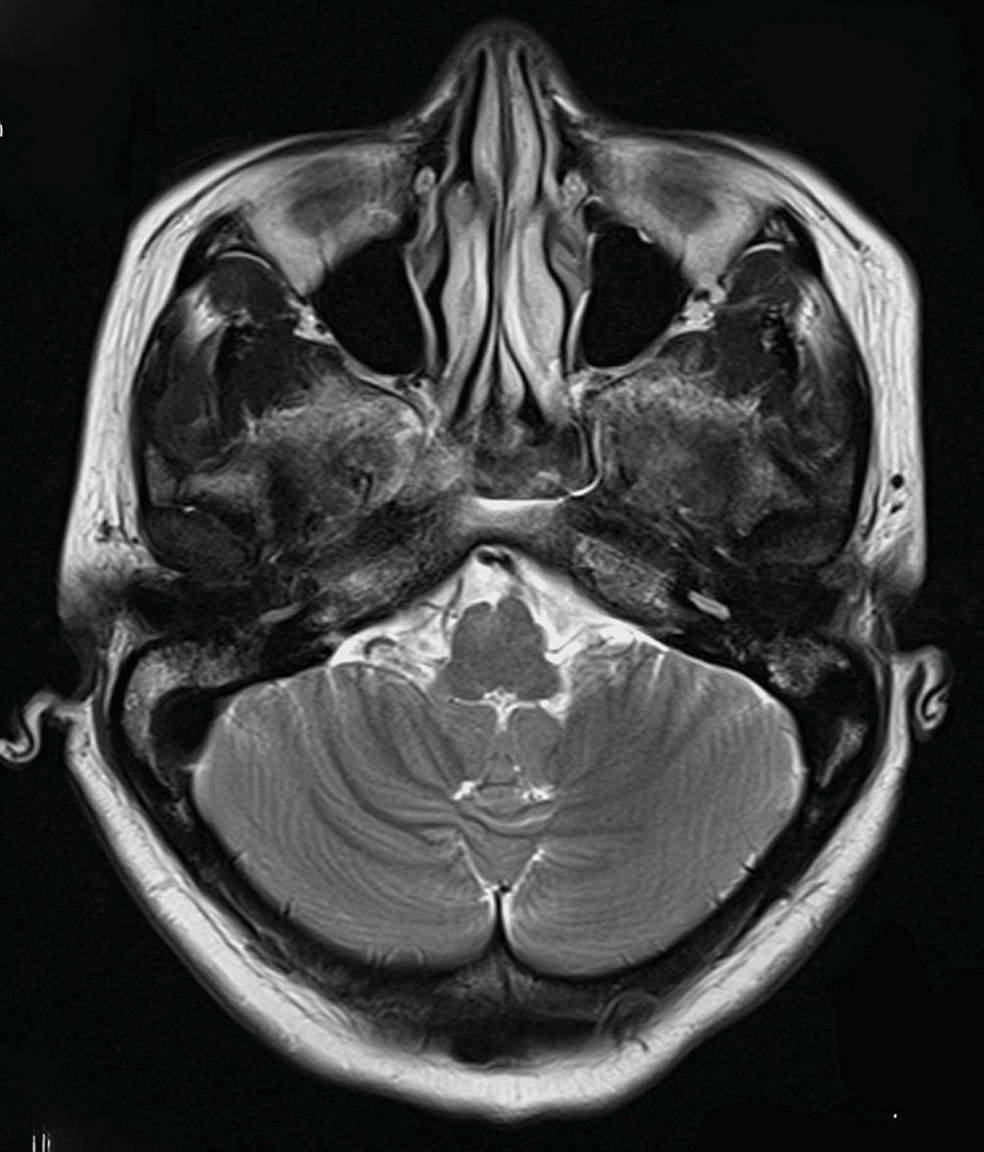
Follow-up magnetic resonance imaging (MRI) brain after two months: normal study
